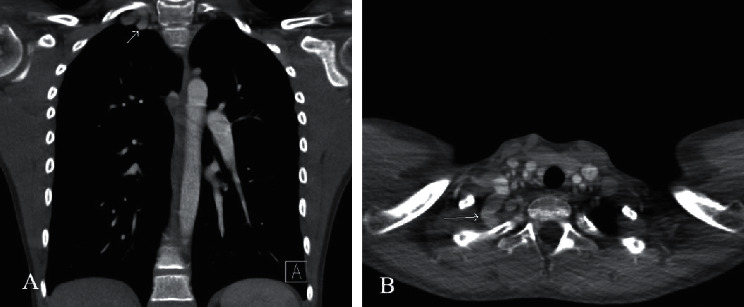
Computed tomography of chest with contrast; coronal (a) view and transverse (b) thin-slice view of the aberrant blood vessel arising from the right brachiocephalic artery, between the right vertebral and right subclavian arteries.
